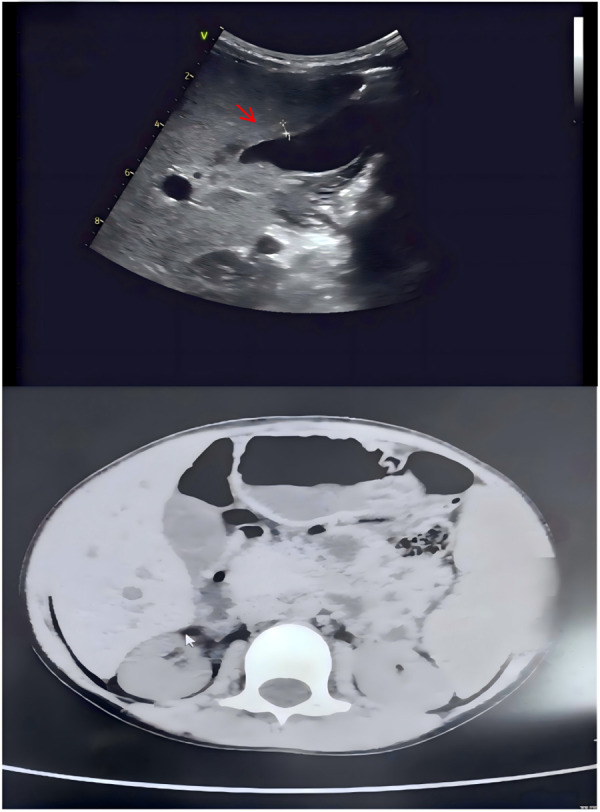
Abdominal ultrasound findings in the acute phase (May 28, 2025). Ultrasound showed marked gallbladder wall thickening with edematous/honeycomb-like change and no gallstones, consistent with acute acalculous cholecystitis. Splenomegaly was also present.
